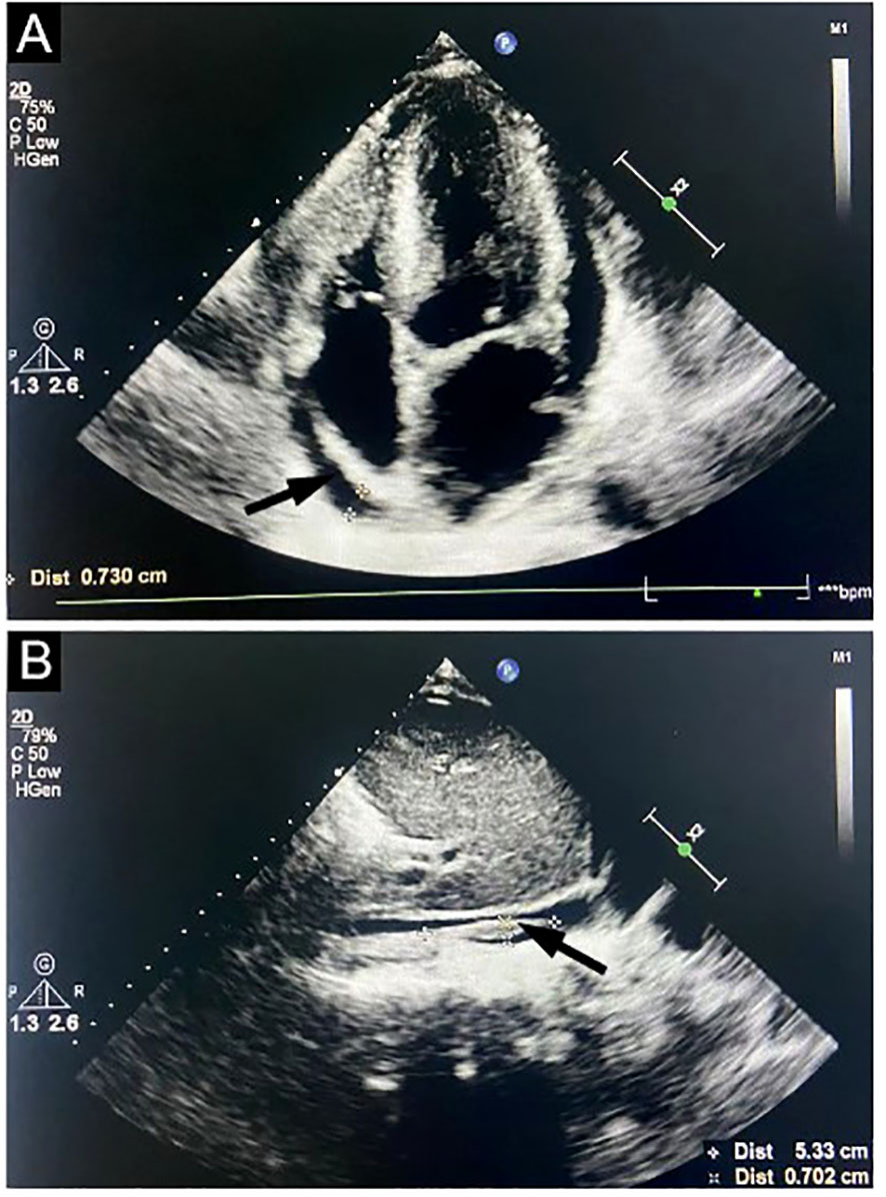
Echocardiography study. Doppler ultrasound images targeting the heart show left ventricular hypertrophy, thickened ventricular septum, enlargement of the left atrium, pericardial effusion (A, arrow), and thrombosis of inferior vena cava near the right atrium entrance (B, arrow).
